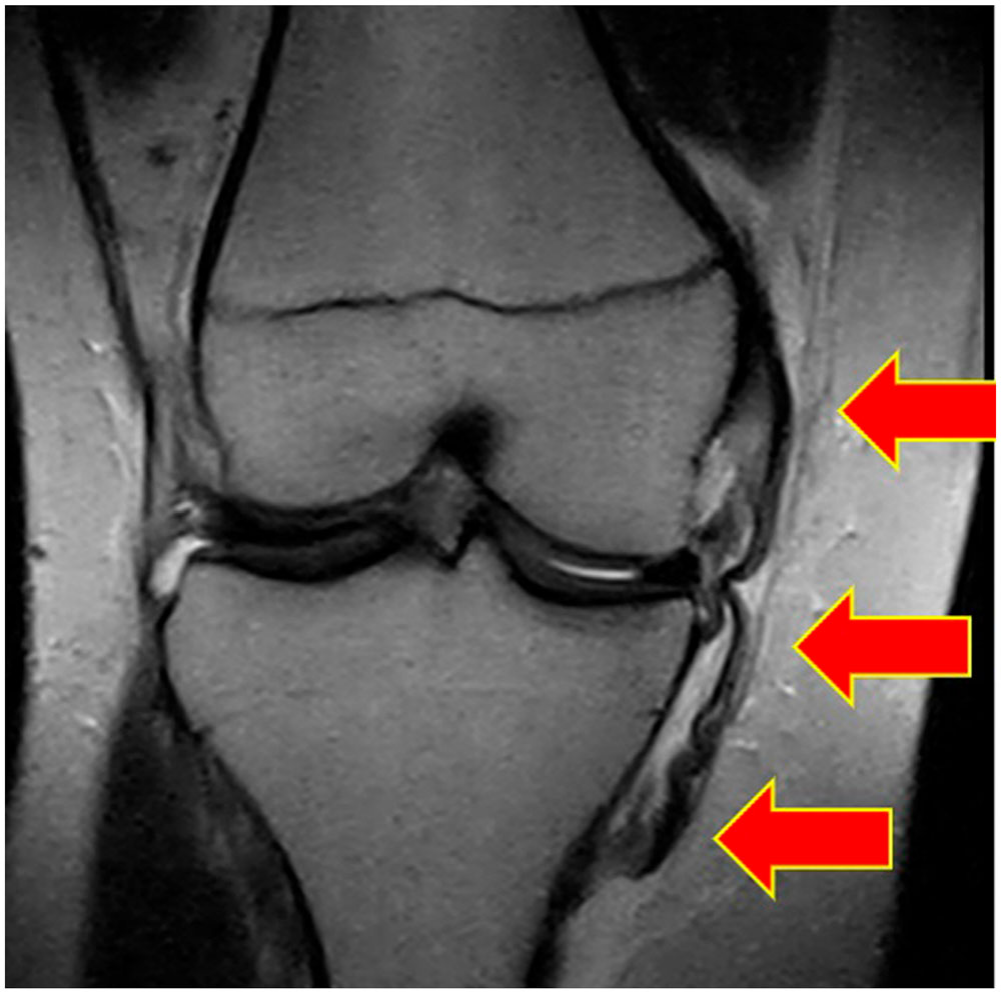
Magnetic resonance imaging, Coronal view. Red arrows show MCL in a right knee with a distal STENER‐like lesion. MCL, medial collateral ligament.
